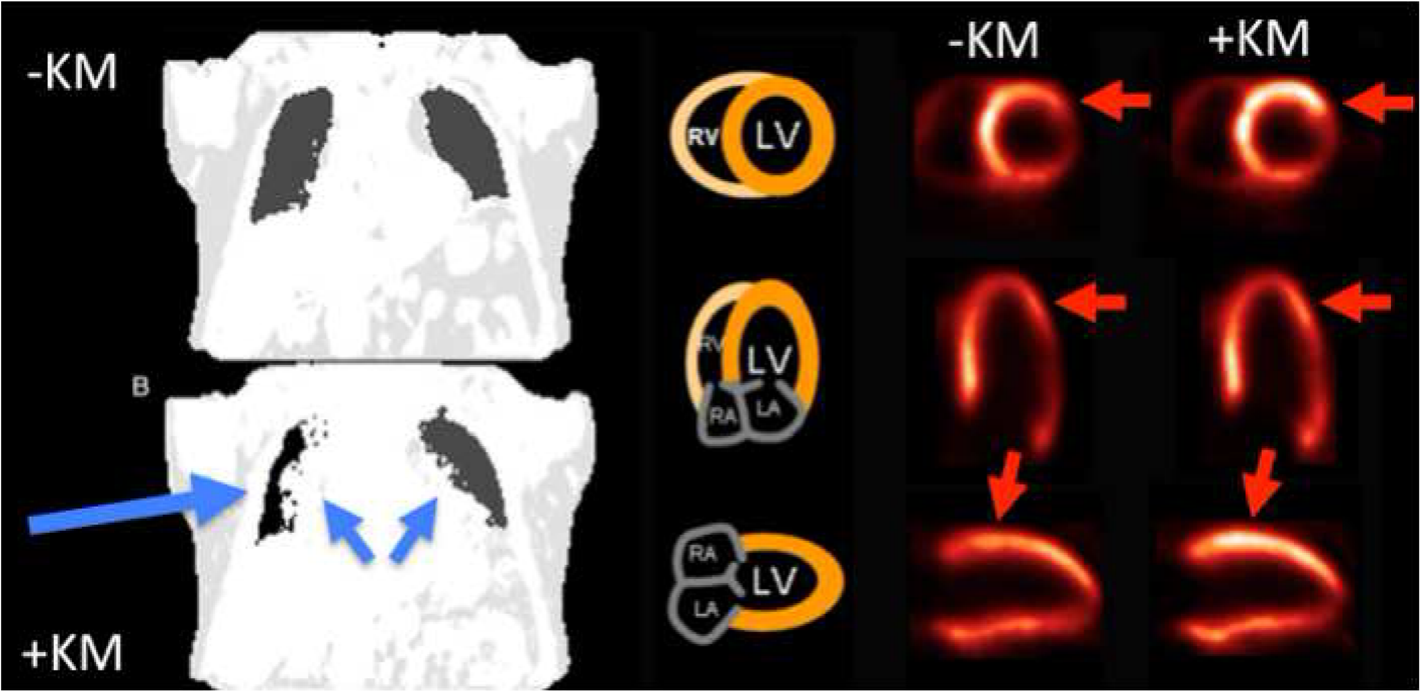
Effects of contrast media on attenuation-correction in PET/MRI. Attenuation correction (AC)-maps derived from Dixon VIBE MR sequences before (upper row, left) and after contrast media application (bottom row, left). Contrast media application may cause erroneous segmentation of the lungs (right lung: air instead of lung tissue indicated by large blue arrow) and in hilar regions (overestimation of soft tissues bihilarly, small blue arrows). This translates into an erroneous tracer uptake increase on attenuation corrected PET images in the anterolateral wall (red arrows). (Reprinted with permission from Rischpler C, Nekolla SG, PET Clin 11 (2016) 465–477 10.1016/j.cpet.2016.05.006)
